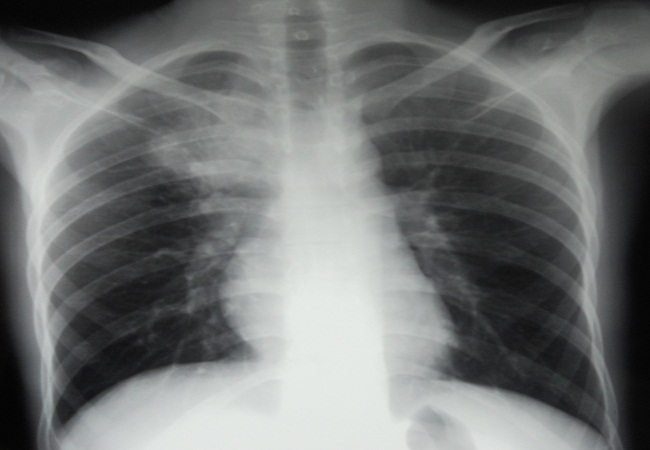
La radiographie thoracique de face objective une opacité hilaire droite hétérogène à contours irréguliers
